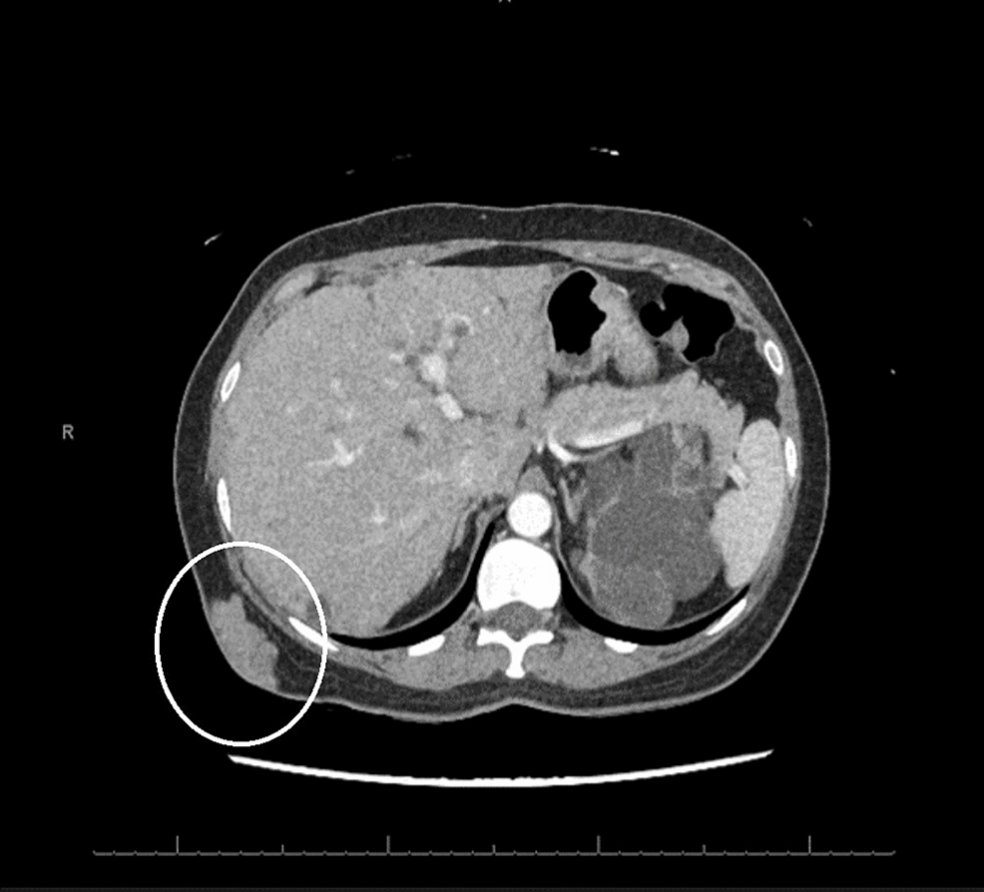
Computed tomography scan with intravenous contrast revealing a soft tissue mass in the subcutaneous fat of the right posterolateral chest wall.
